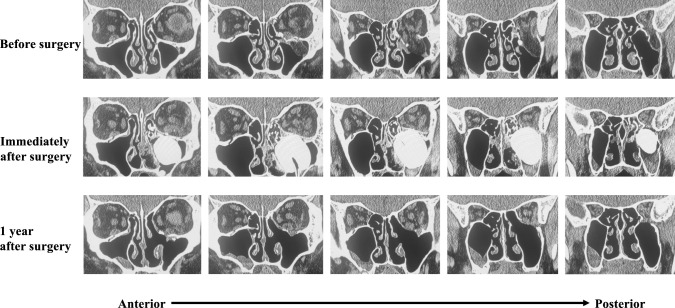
Comparison of computed tomography (CT) images before surgery, immediately afterward, and 1 year afterward. The upper, middle, and lower sections show CT images before surgery, immediately afterward, and 1 year afterward, respectively. The most anterior slices are shown on the left, and the most posterior slices are shown on the right. The orbit is well repaired 1 year after surgery
